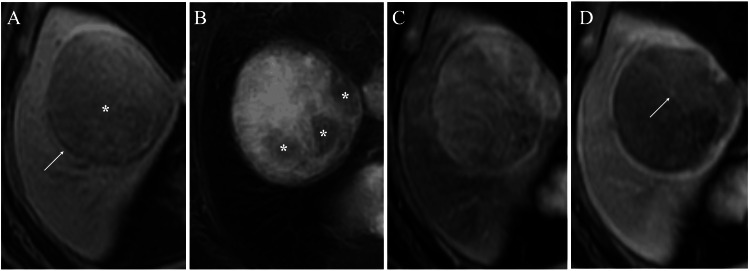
Magnetic resonance imaging (MRI) findings (A) The T1-weighted image showed an oval mass (asterisk) and the surrounding hypointense ring (arrow). (B) The fat-suppressed T2-weighted image showed that the mass had a mixed hypointense (asterisks) and weak hyperintense areas. (C) Subtraction image at 39 seconds after the contrast medium injection showed a weak early enhancement of the mass. (D) Subtraction image at 120 seconds after the contrast medium injection showed a wash-out pattern with residual slight enhancement of the mass (arrow).
